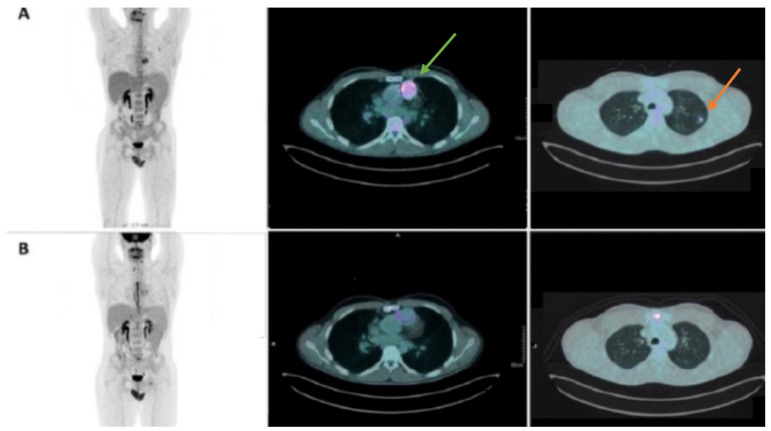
(A) (MIP and fused axial [^18^F]FDG PET-TC images): [^18^F]FDG PET images show increased radiopharmaceutical uptake around the aortic valve prosthesis (revealing an infective process, positive for Granulicatella adiacens; green arrow) associated with a lung septic embolism (orange arrow). An adequate preparation before PET imaging (consisting of a very low carbohydrate, high protein, and high-fat diet the day before, followed by fasting overnight on the day before imaging) allows for minimizing physiological 18F-FDG myocardial uptake. (B) (MIP and fused axial [^18^F]FDG PET-TC images): [^18^F]FDG PET images show no pathological uptake after surgical stent removal (as after surgical procedure) and medical treatment (with complete clinical response for lung embolism).
